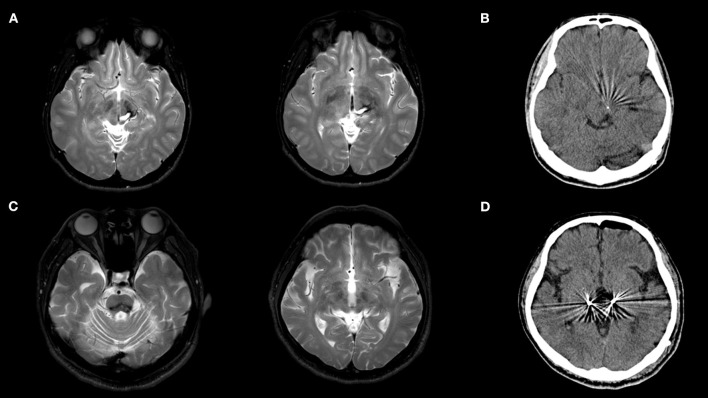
(A) Brain MRI showed an old hemorrhage in patient 1. (B) Postoperative CT showed the electrode was precisely implanted in the PSA in patient 1. (C) Brain MRI showed the old hemorrhage in the pons in patient 2. (D) Postoperative CT showed the electrodes were precisely implanted in the PSA in patient 2.
